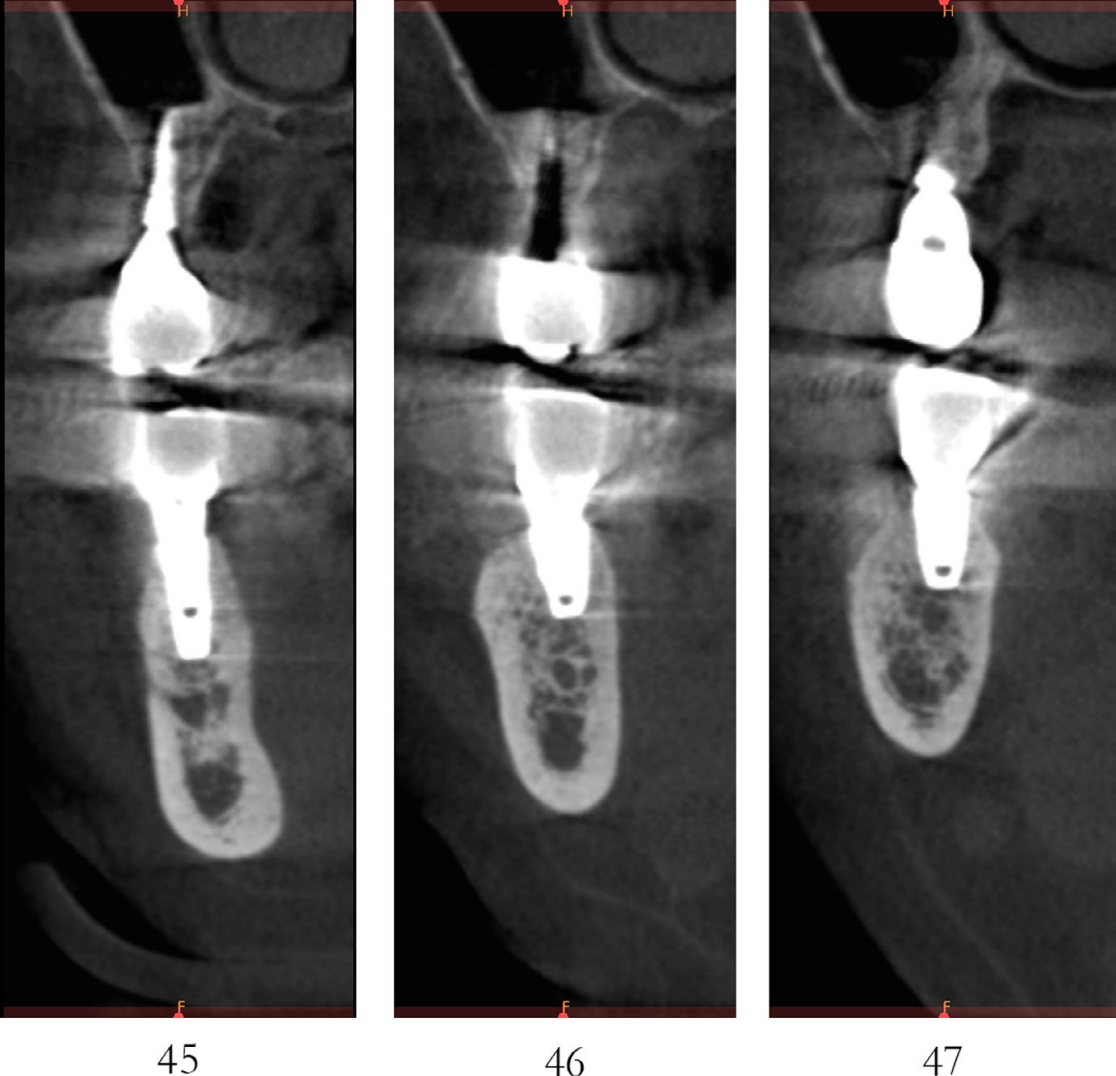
CBCT scanning 2 y after final restoration
